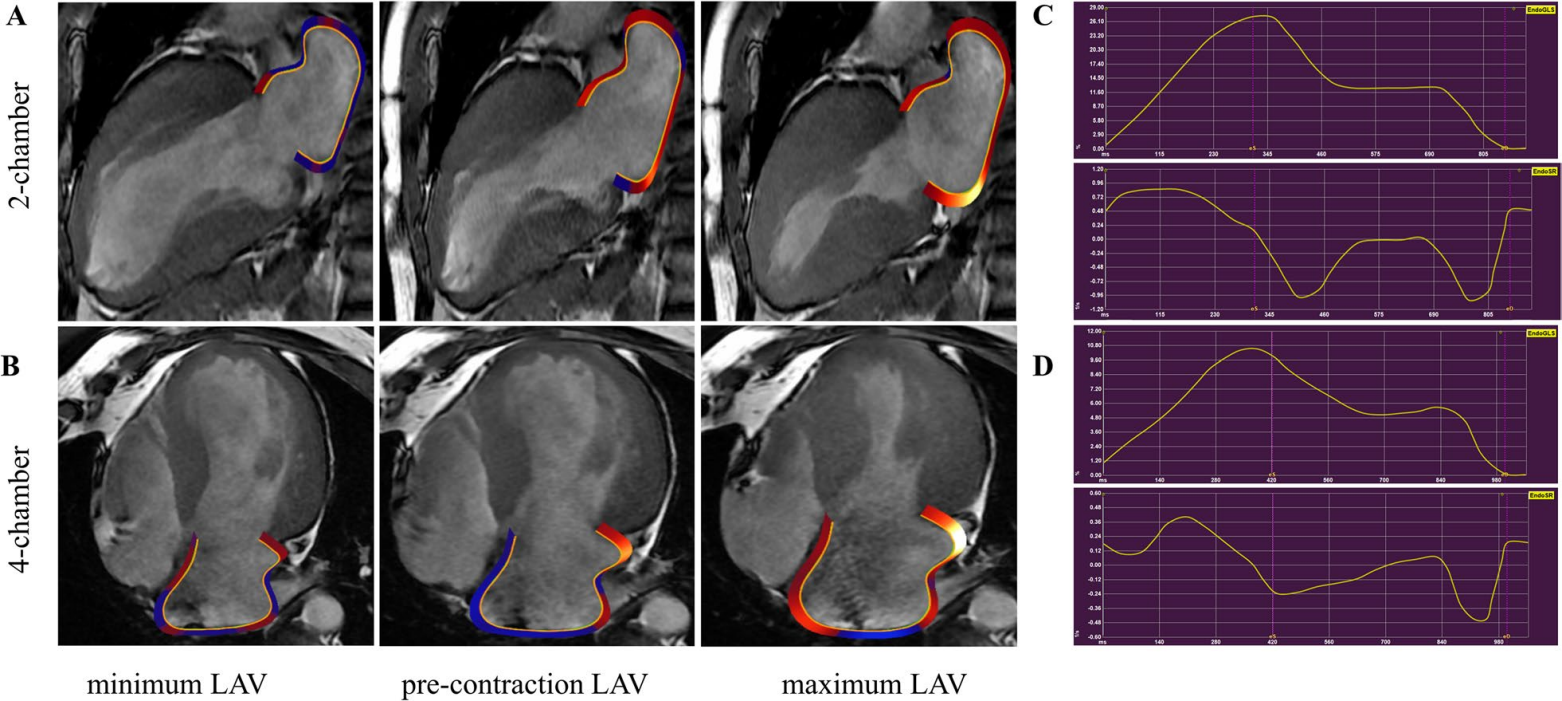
LA tracing in cine cardiac MRI A two-chamber and B four-chamber views. C LA GLS and SR curves for HCM patients without combined adverse clinical events; D LA GLS and SR curves for HCM patients with combined adverse clinical events. LA, left atrium; GLS, global longitudinal strain; SR, strain rate
